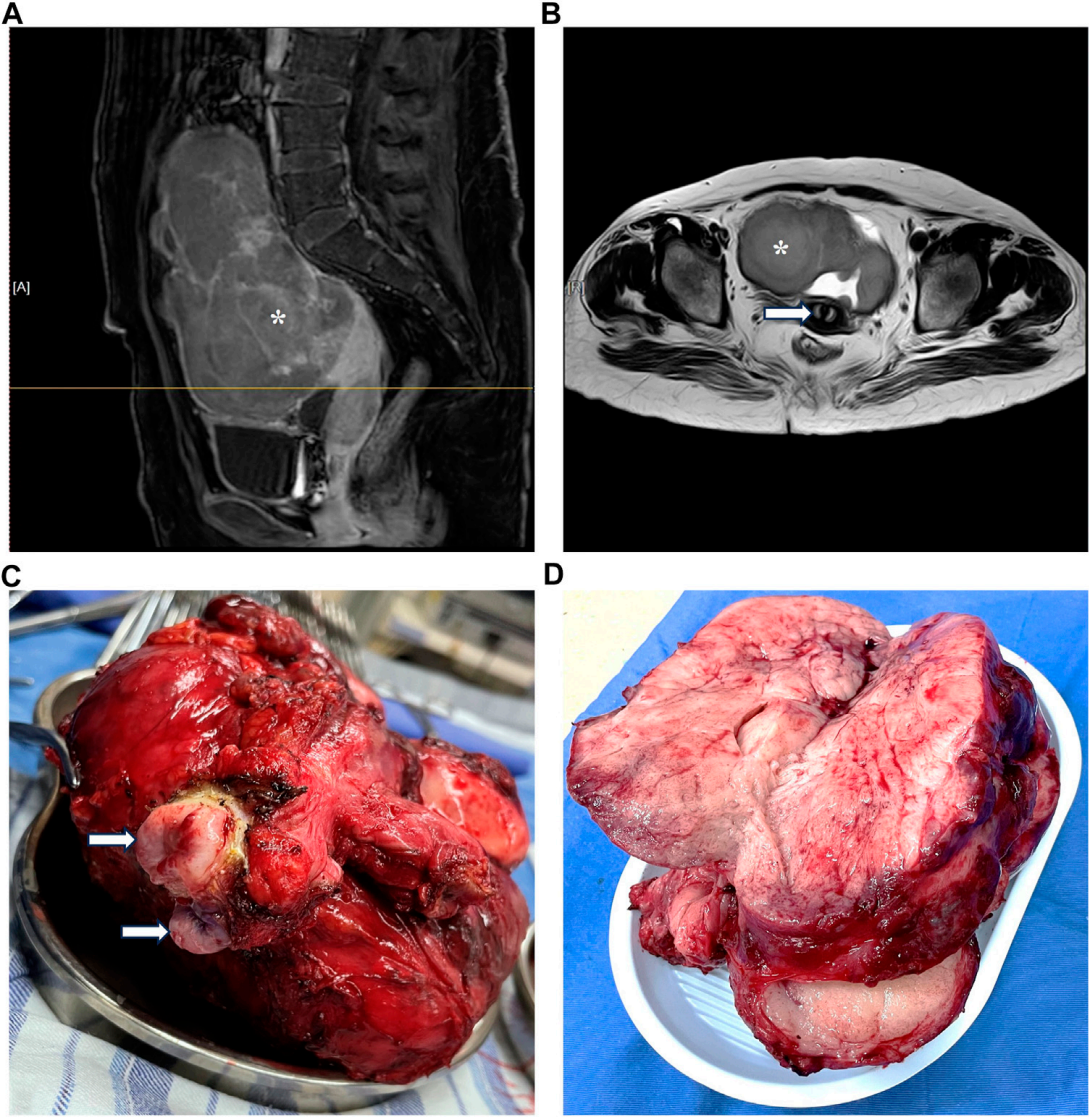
Magnetic resonance imaging and radical surgery.
